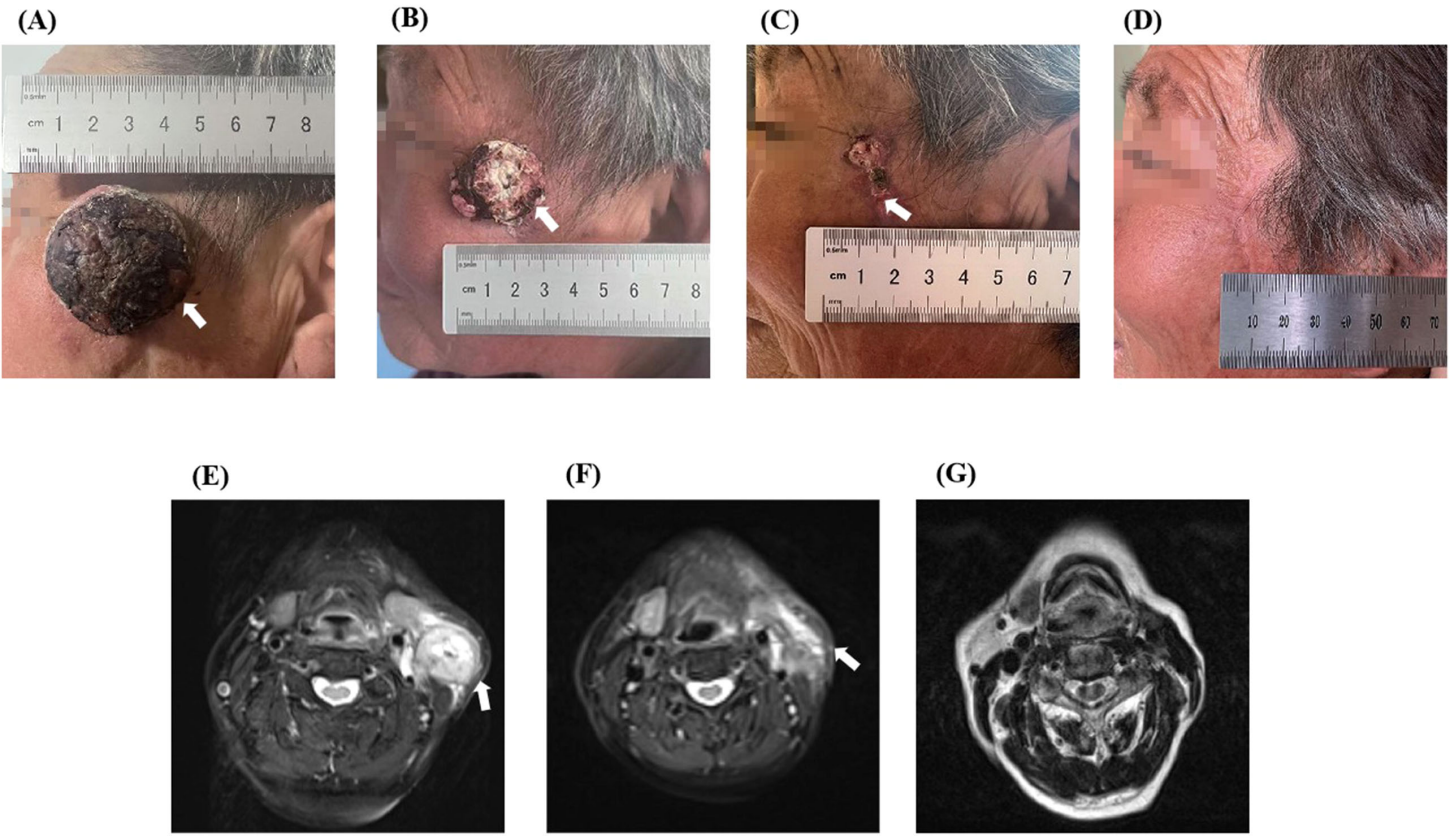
The comparison images of before and after treatment of the patient. Upon admission, a mass of about 3.5 cm × 3.5 cm was visible in the patient’s left temporal region, neck, and MRI showed abnormal signals in the shadow of the neck mass (A, E). After one cycle of treatment, the quality was significantly lower than before treatment, and repeated MRI showed that the shadow quality of the neck was lower than before treatment (B, F). (C) shows a visual view of the patient’s left temporal tumor after two cycles of treatment, leaving only postoperative scabs. (D) shows the visual observation of the patient after one year of treatment, leaving only postoperative scars, and (G) shows the MRI images of the patient’s neck after one year of treatment.
